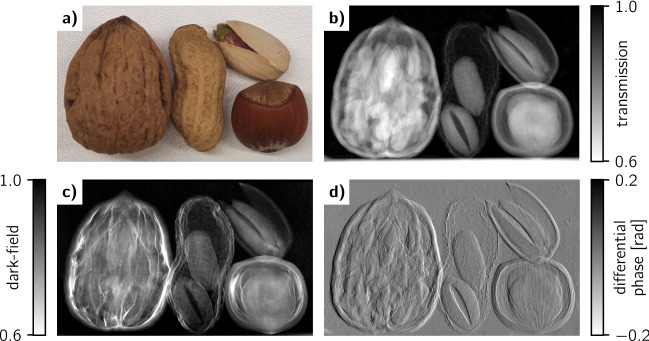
Imaging example using the fabricated W particle grating as analyzer grating in an X-ray grating interferometer. (a) Photograph of the imaged nuts, namely a walnut, a peanut, a pistachio, and a hazelnut. (b) Transmission image, (c) dark-field image, and (d) differential phase image of the four nuts acquired at 60 kV tube voltage.
